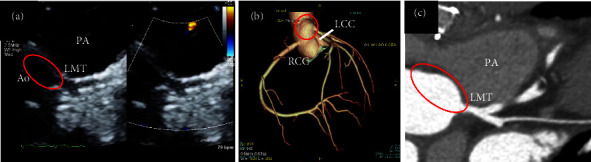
Transthoracic echocardiography (a) and computed tomography angiography (b, c) 6 months after surgery showing the new left coronary ostium with unroofing of the intramural portion and patch enlargement using the great saphenous vein graft (red circles). Ao: aorta; PA: pulmonary artery; LMT: left main coronary artery trunk; LCC: left coronary cusp; RCC: right coronary cusp.
